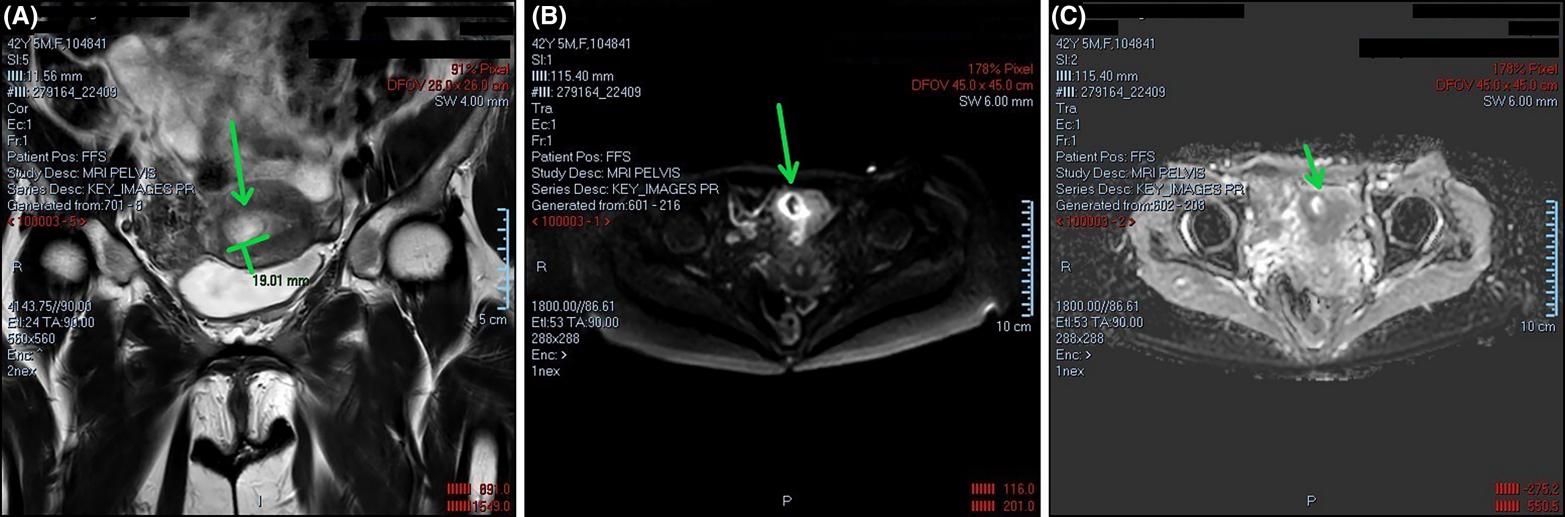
Findings from the MRI scan performed during the 7th week of gestation. The scan verified the diagnosis of cornual pregnancy (A) coronal plane, (B, C) transverse plane.
